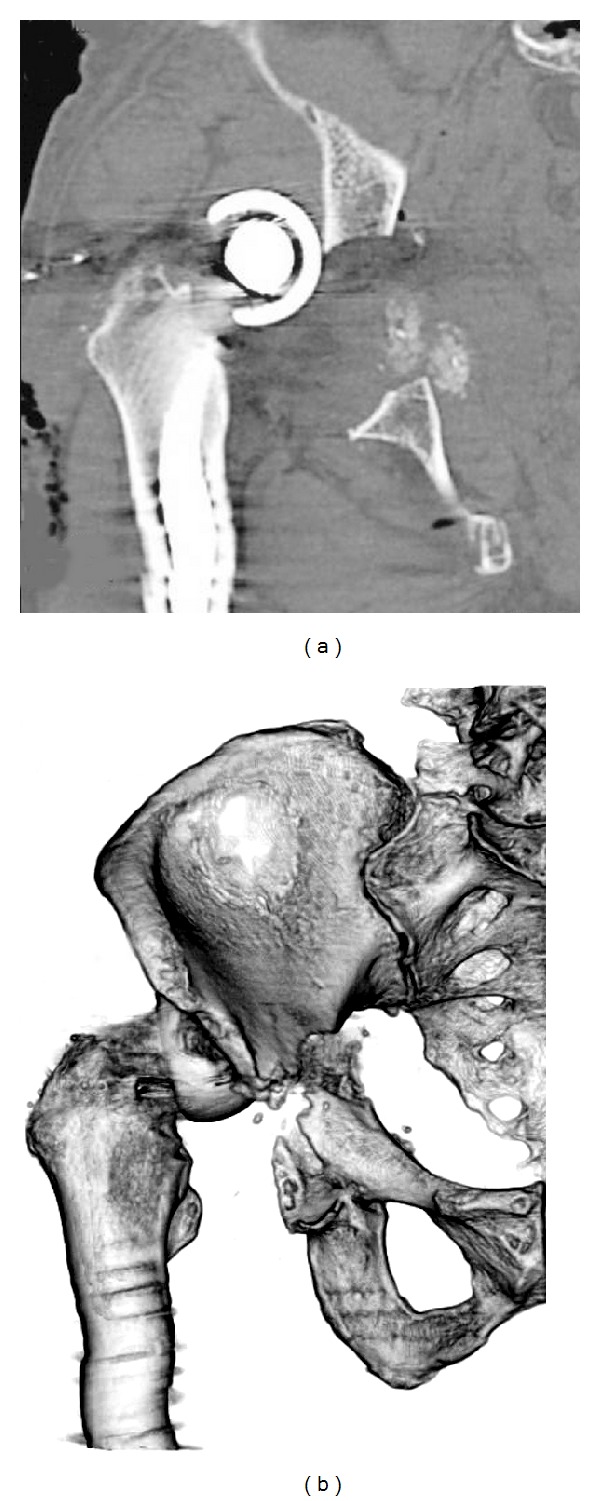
MDCT images showing pelvic discontinuity. (a) Coronal multiplanar reconstruction computed tomography. (b) 3D image in the anteroposterior view.
